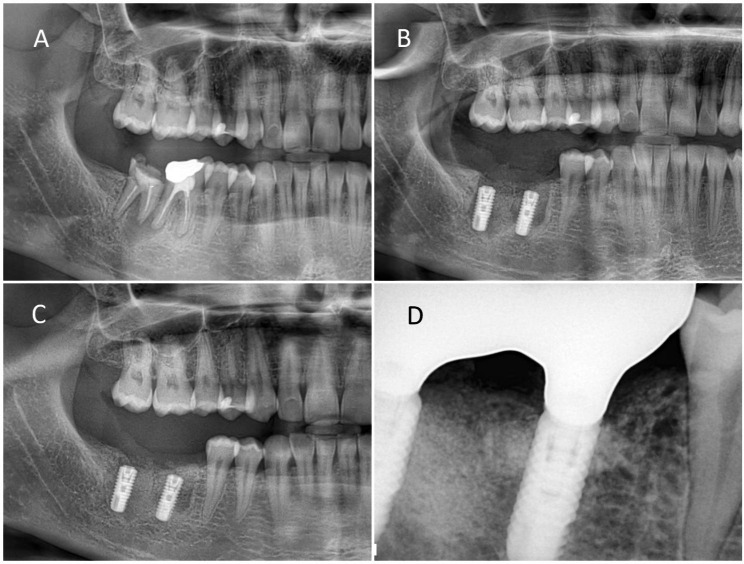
(A) Right mandibular first and second molars with chronic periapical pathology due to unsuccessful endodontic treatments. (B) Extraction of teeth, placement of immediate implants and filling of socket cavities with guided bone regeneration (1st postoperative week). (C) Postoperative 5th month panoramic radiograph image. (D) Follow-up radiograph at 12 months after completion of prosthetic restorations
